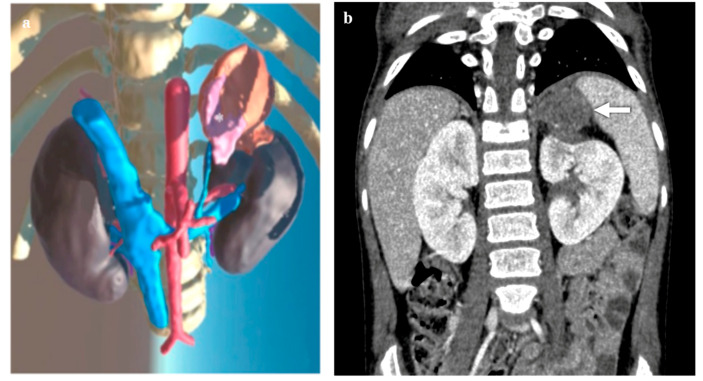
Virtual reality (VR) (a) and coronal computed tomography (CT) (b) images of a 4-year-old girl with left adrenal neuroblastoma (3.5 × 2.5 × 4 cm in diameter) (* in a; → in b) in contact with the upper third of the left kidney and the spleen; images show the adrenal vein and the impressions of the adrenal mass on surrounding organs.
